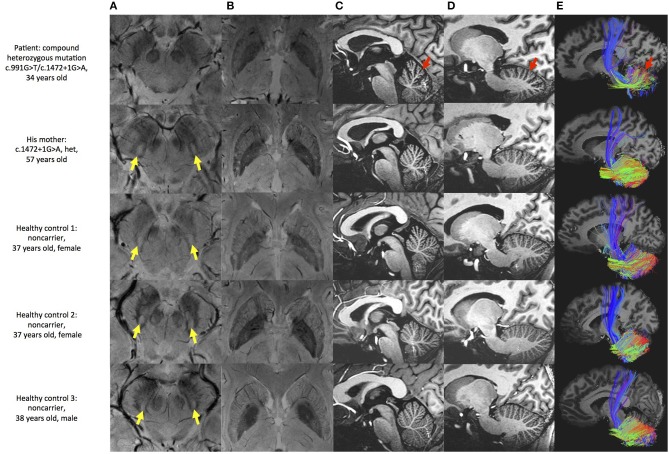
Brain MRI examination of the patient and controls. (A) SWI image shows substantia nigra and red nucleus. Hyperintensity is found in substantia nigra of patient's mother and healthy controls (yellow arrow), but not in this patient. (B) SWI image shows basal ganglia. Hypointensity can be seen in globus pallidus and margin of putamen in all subjects. (C,D) MP2RAGE sagittal image shows deepened cerebellar fissures in this patient (red arrow). (E) Fiber tracking shows sparser tract in the patient (red arrow).
